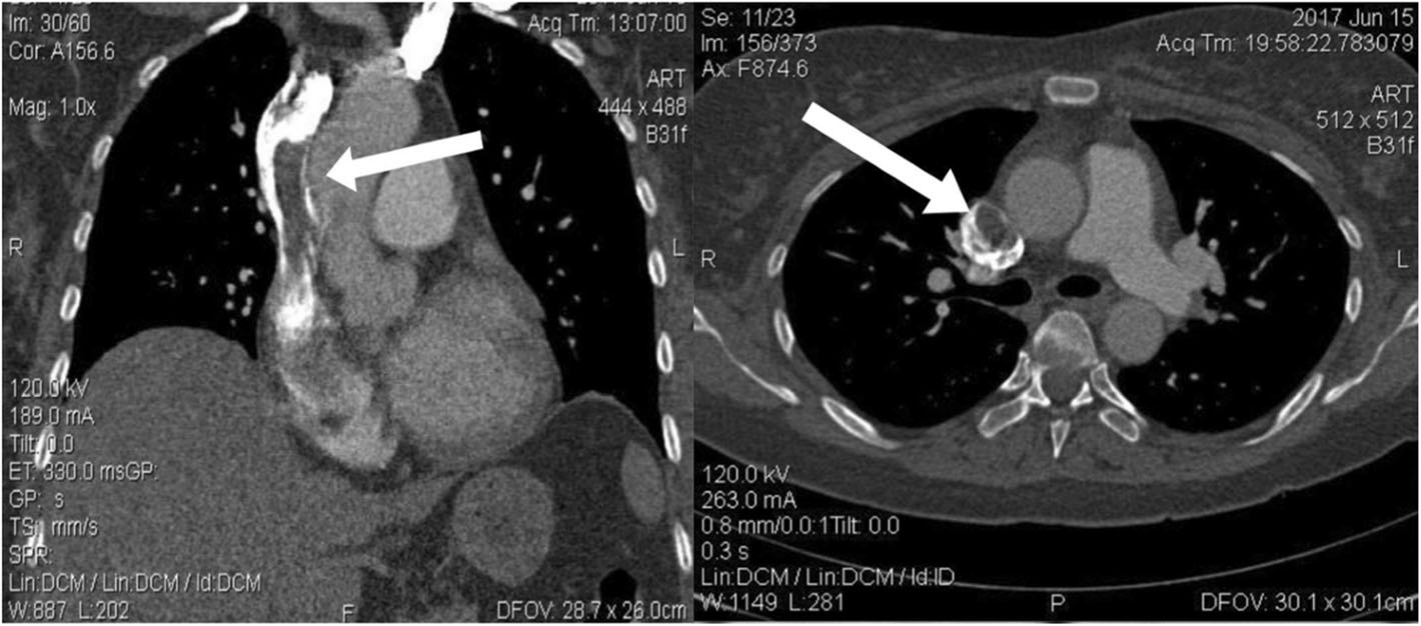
Computed tomographic angiography demonstrating a filling defect in the superior vena cava. The arrow is pointing to these filing defects
